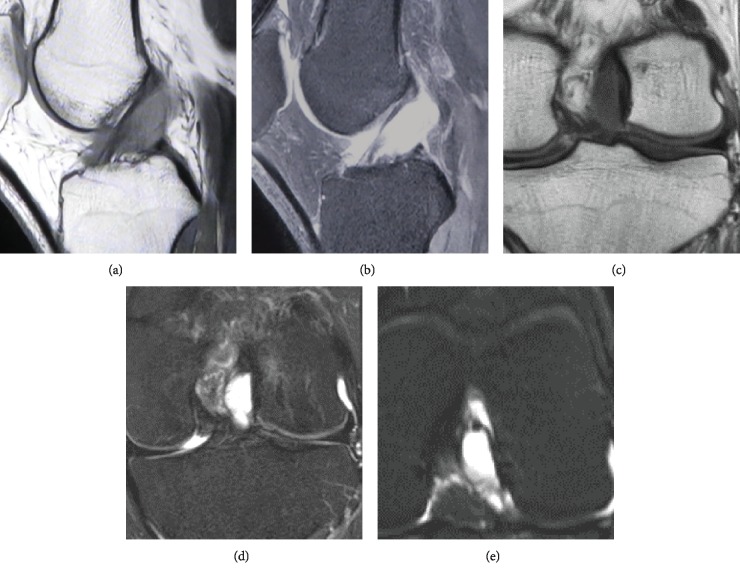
Magnetic resonance images obtained preoperatively. MRI revealed thinning of the ACL and a cystic lesion (*φ*23 × 12 mm) in the intercondylar fossa that was hypointense on T1-weighted imaging and hyperintense on T2-weighted imaging. (a) Sagittal T1-weighted scan. (b) Sagittal T2-weighted scan. (c) Coronal T1-weighted scan. (d) Coronal T2-weighted scan. (e) Axial T2-weighted scan.
